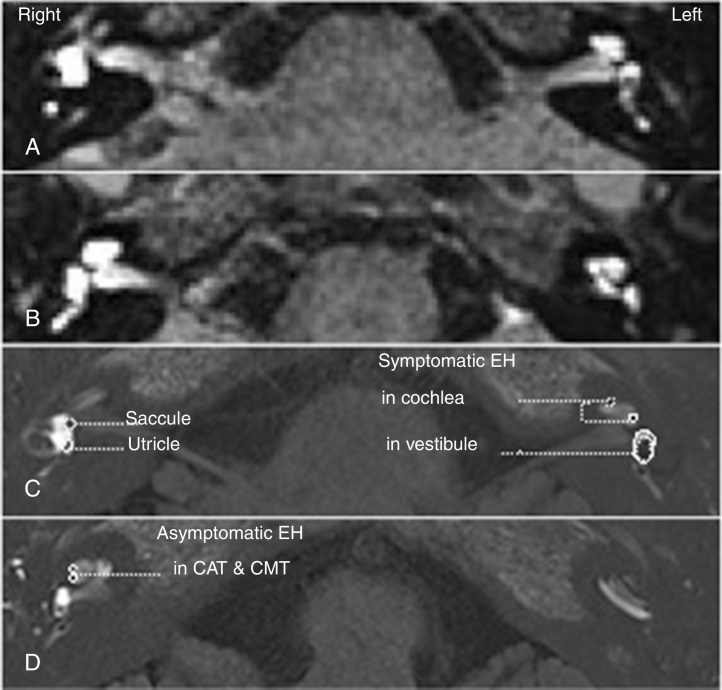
Bilateral endolymphatic hydrops (EH) was detected in a patient diagnosed with left unilateral Meniere's disease (MD). Three-dimensional fluid-attenuated inversion recovery (3D-FLAIR) (A, B) and three-dimensional real inversion recovery (3D-real IR) (C, D) magnetic resonance imaging (MRI) was performed 24 hours following a bilateral intratympanic gadolinium (Gd) injection. 3D-FLAIR MRI showed bilateral perilymphatic space permeated with Gd (A, B). EH was present in bilateral cochleae (C, D) and the left vestibule (A, C), but absent in the right vestibule (B, C, D). Symptomatic EH was present in all turns of left cochlea, while asymptomatic EH was only present in apical and middle turn (CAT & CMT) (C, D). The degree of symptomatic EH in the left cochlea (affected side) was greater than that of the asymptomatic EH in the right cochlea (unaffected side) (C, D). Significant EH was observed in the left vestibule, in which the boundary between the saccule and the utricle could not be detectable (C). The saccule and utricle could be detected respectively in the right vestibule, in which no EH was present (C).
